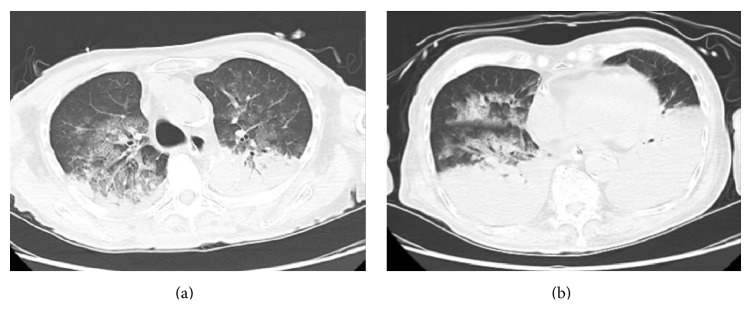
A thoracic computed tomography scan demonstrated bilateral parenchymal consolidation in gravity-dependent areas and ground glass-appearing opacities of lung parenchyma (a). Bilateral atelectasis was observed in the lower lung fields (b).
